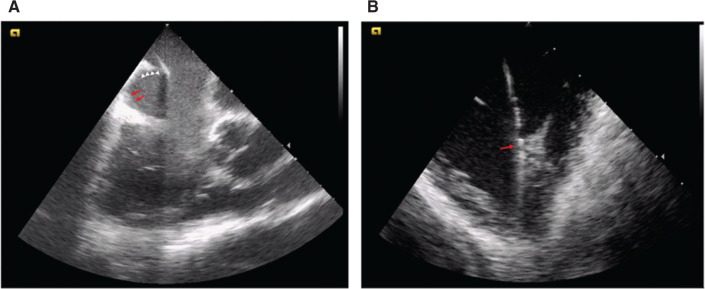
Intracardiac ultrasound can be an indispensable tool in navigating complex atrial and ventricular anatomy. A: Prominent Eustachian ridge (white arrowheads) impeding catheter access to the cavotricuspid isthmus and requiring a “candy cane” approach with the ablation catheter (red arrow). B: Patient with symptomatic premature ventricular complexes (PVCs) that were only terminated by ablation at the bifurcation of the right ventricular papillary muscle (red arrow) seen with intracardiac echocardiography. Ablation lesions delivered on either site of the structure led to partial suppression but not complete elimination of the PVC.
